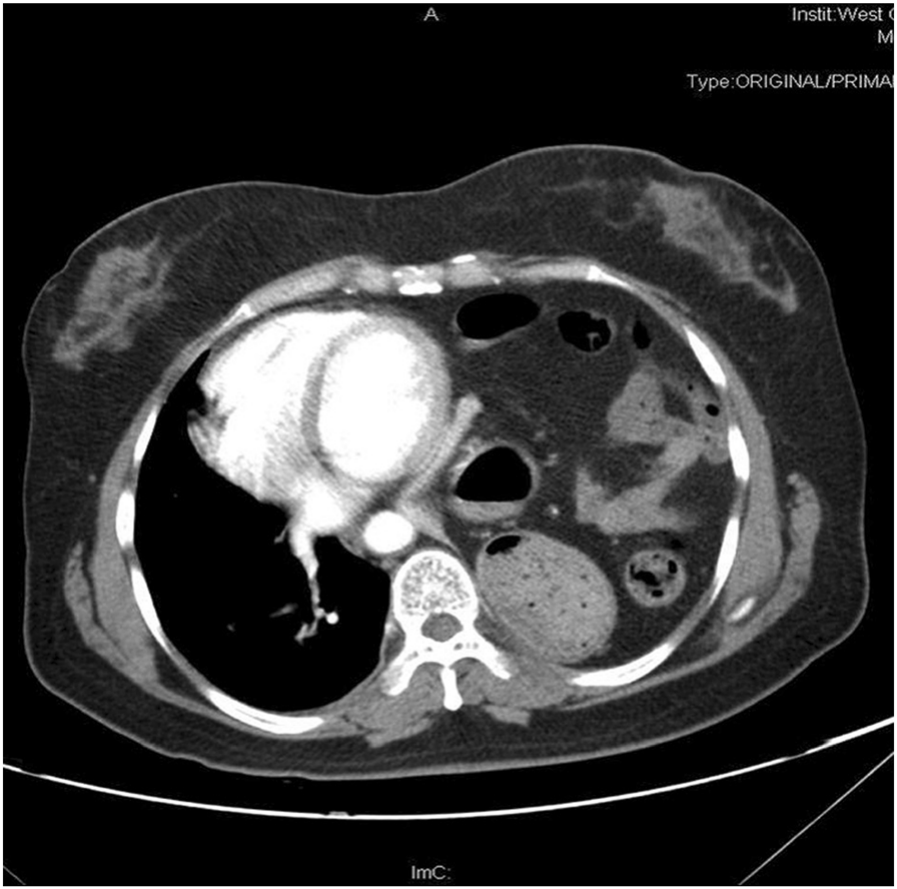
Contrast-enhanced CT scans showed the mediastinum shifted to the right thoracic cavity.
